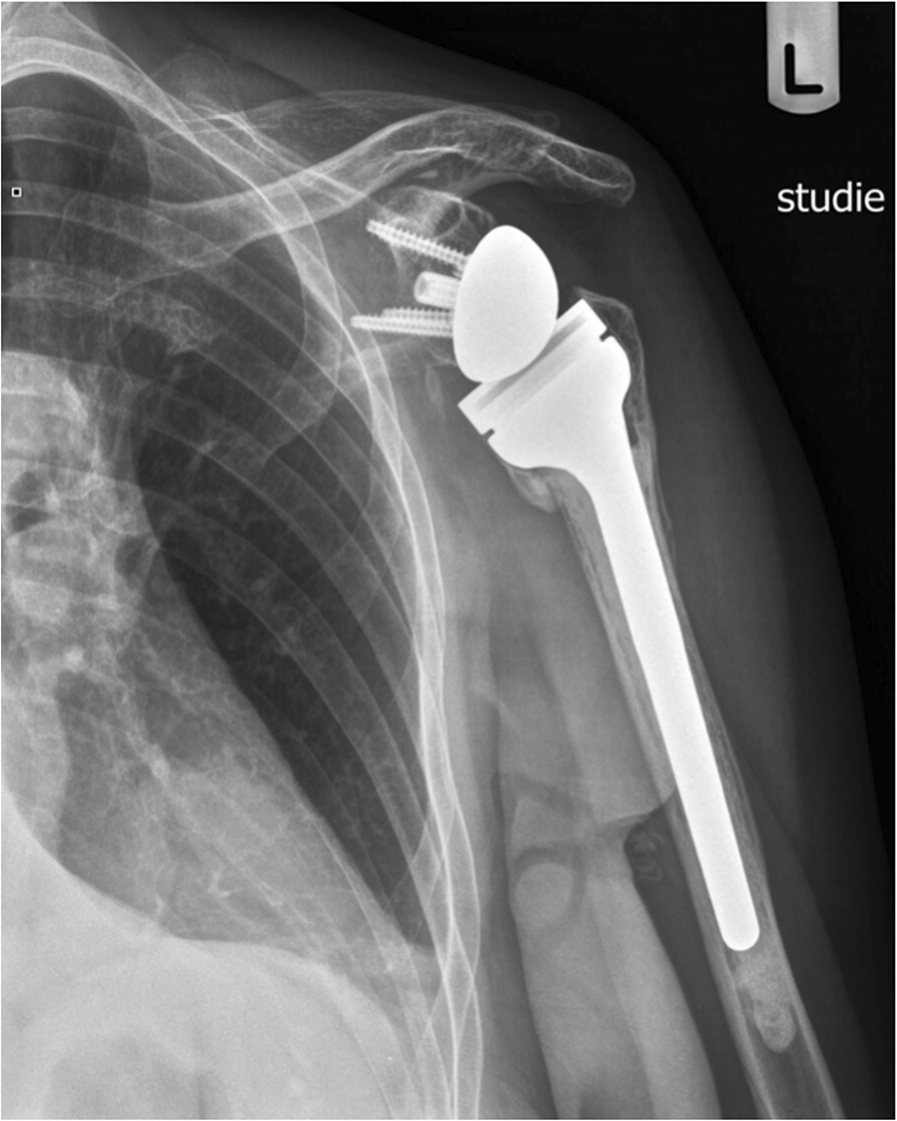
X-ray one year after implantation of reverse shoulder arthroplasty with healed greater tuberosity (a.-p.-view).
